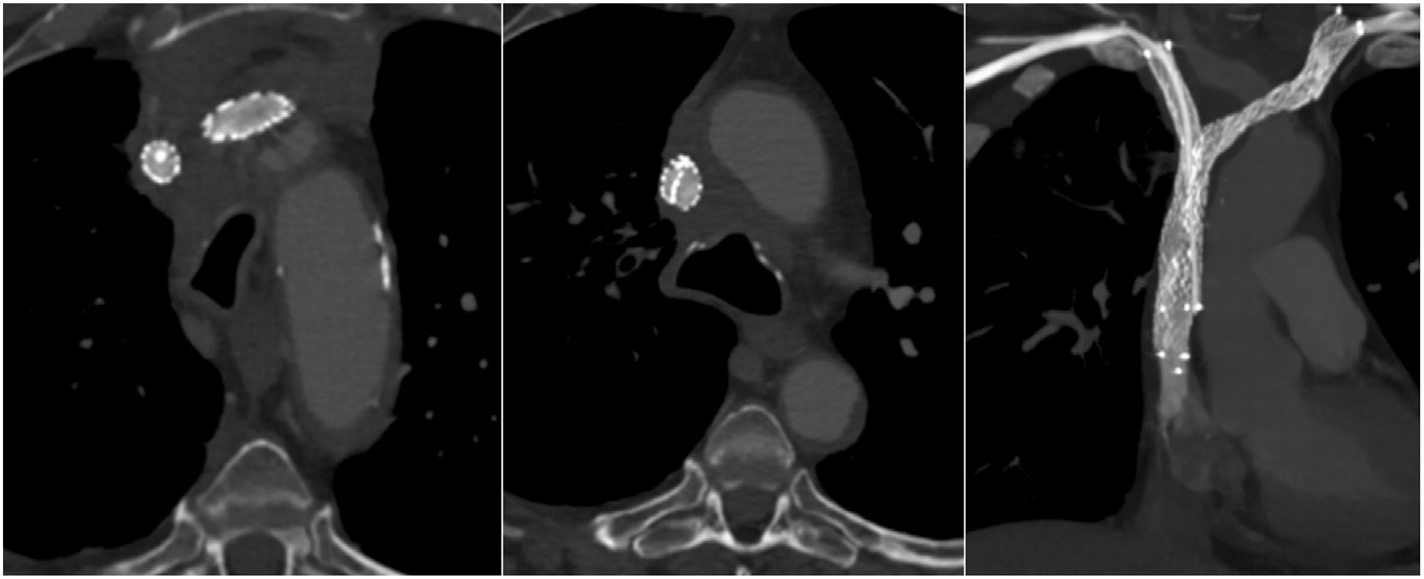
A 3-month follow-up computed tomography (axial sections and coronal section in maximum intensity projection) demonstrated bilateral stents patency without collateral veins. The left-sided stent, which ensures head and neck venous drainage, had a larger expansion than the right-sided one.
